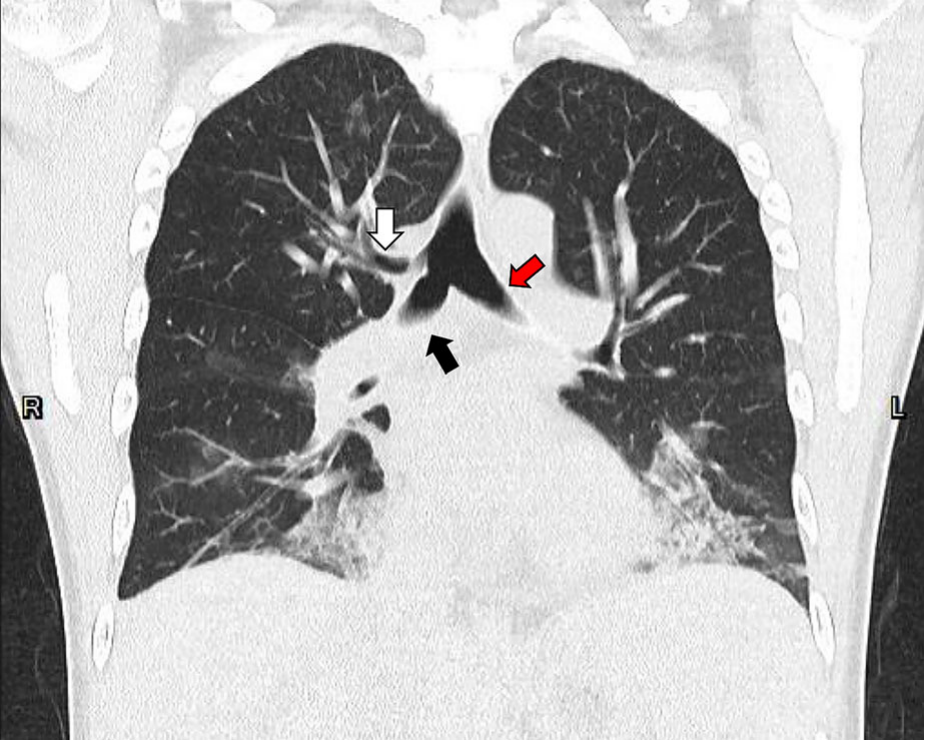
Coronal view of the chest computed tomography with the tracheal bronchus (white arrow), right main bronchus (black arrow) and left main bronchus (red arrow).
